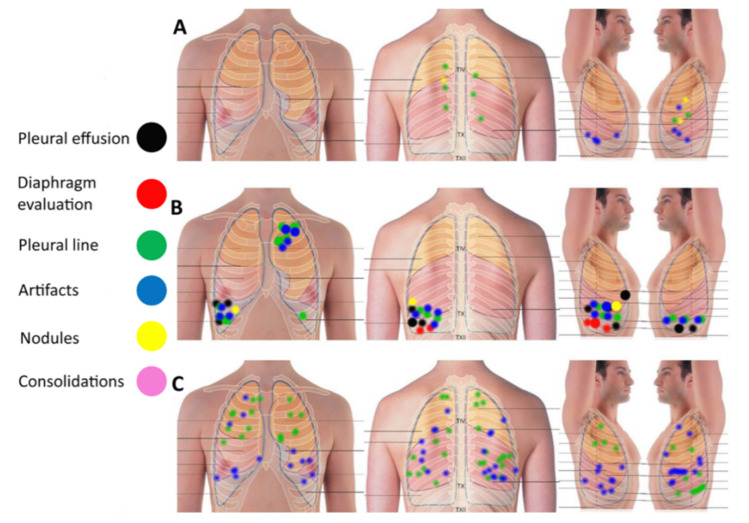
Example of the location of ultrasound (US) findings on the thoracic surface through a graphic scheme with differently colored landmarks, to obtain a superficial topography comparable to CT images. Three schemes of three different patients are shown in A, B and C.
